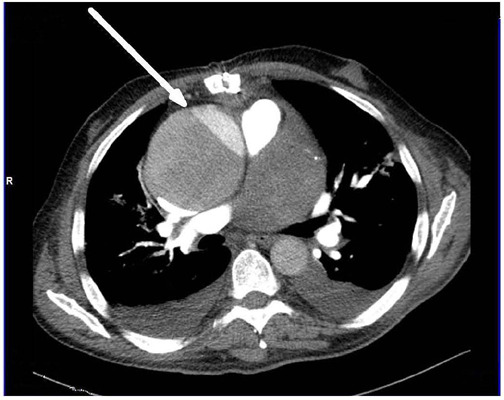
Preoperative 64 multislice computed tomography of the chest. Arrow
